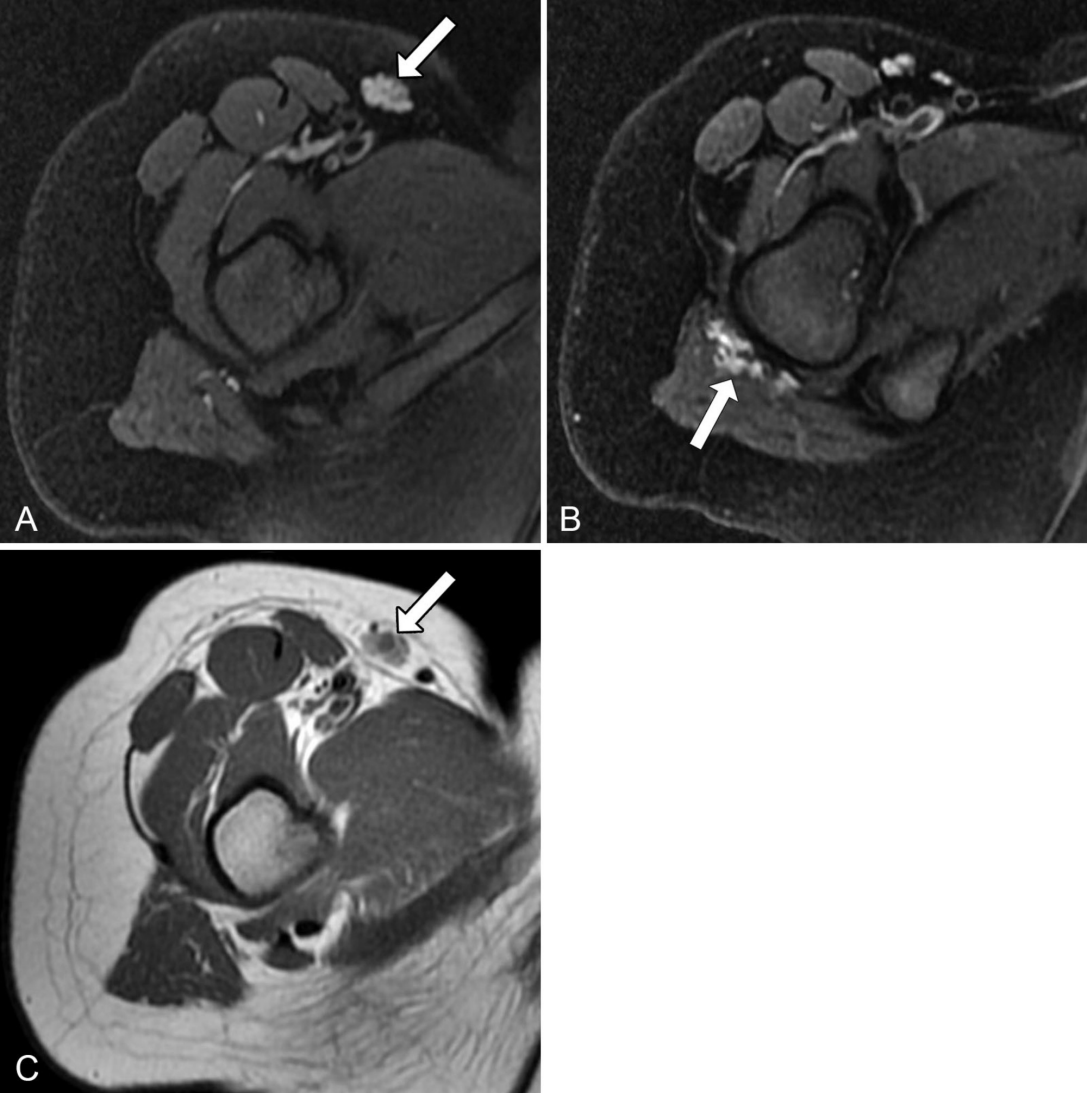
Similar-appearing lesions in the right inguinal subcutaneous soft tissues and gluteus maximus muscle belly (A, B) Axial proton density fat-saturated images demonstrate additional lesions (arrows) in the right inguinal subcutaneous soft tissues and gluteus maximus muscle belly with similar signal characteristics compared to the lesion in the popliteal fossa. However, (C) T1-weighted axial image demonstrates that, unlike the lesion in the popliteal fossa, the lesion in the right inguinal subcutaneous soft tissues (arrow) has a high intrinsic T1 signal.
